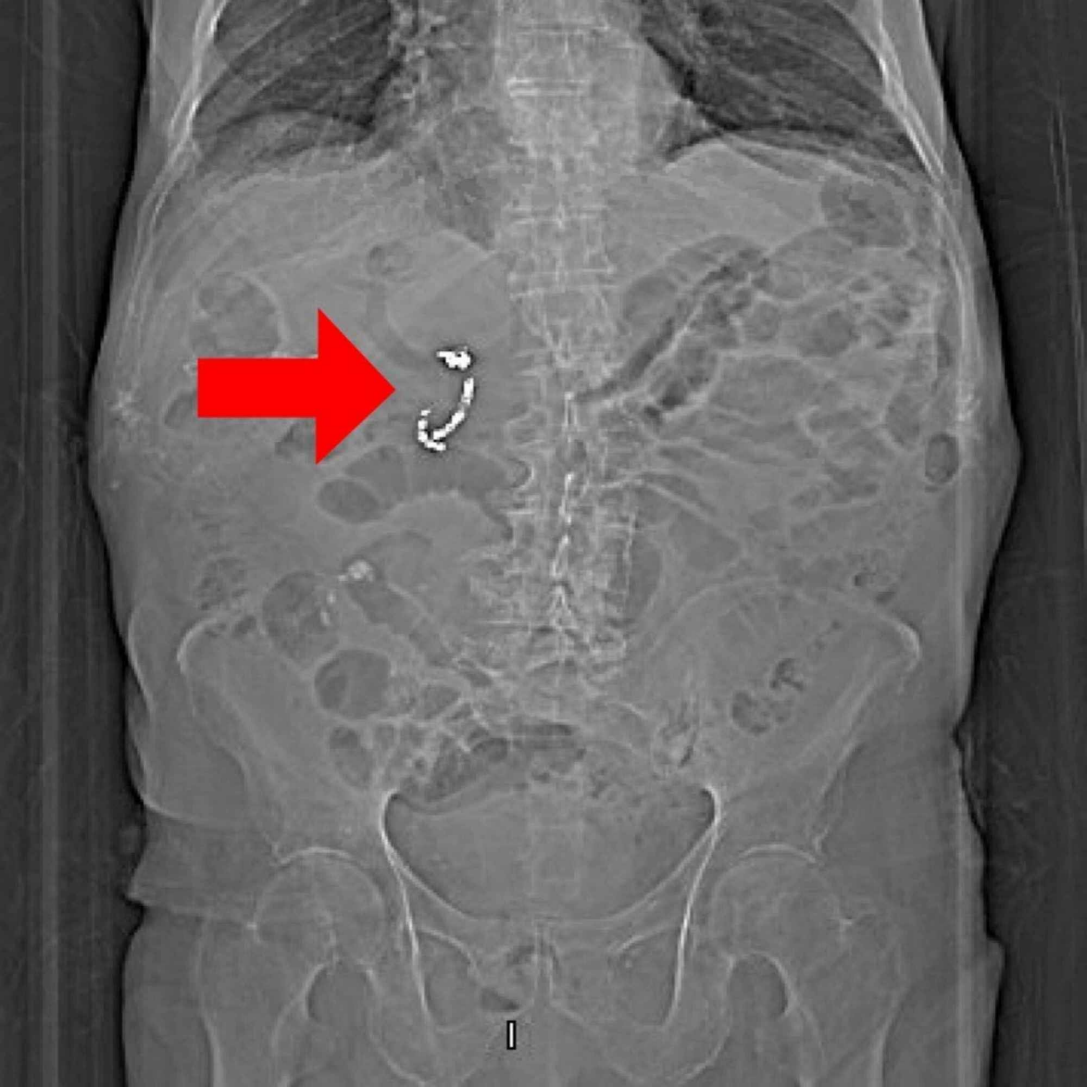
Vascular coil: radiolographical image Abdominal computed tomography showing a vascular coil
